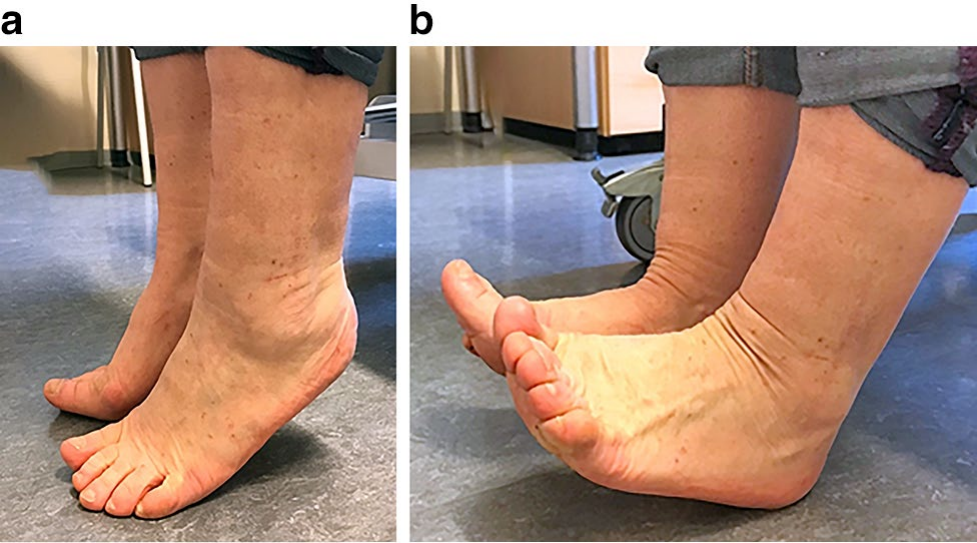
Free function (50 degrees of sagittal ankle motion on both sides) at 69-month follow-up (OMAS 100; AOFAS 100; FFI-D 1.85; SF-36 PCS 54.7 MCS 52.6) of the same patient shown in Figs. 1 and 4. Because the patient was pain free, no indication was seen for another set of radiographs at the time of follow-up
